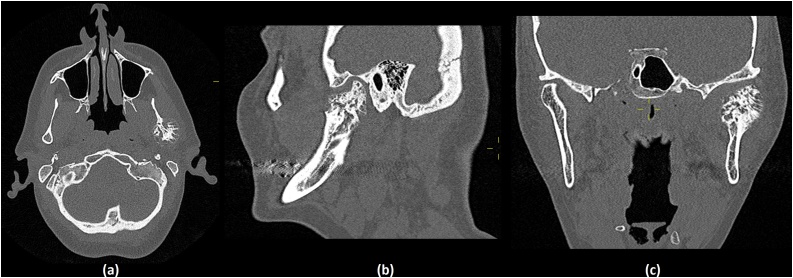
CT Imaging demonstrating the lesion of the left temporomandibular joint and mandible. (a) - Axial plane, (b) - Sagittal plane, (c) - Coronal plane.
